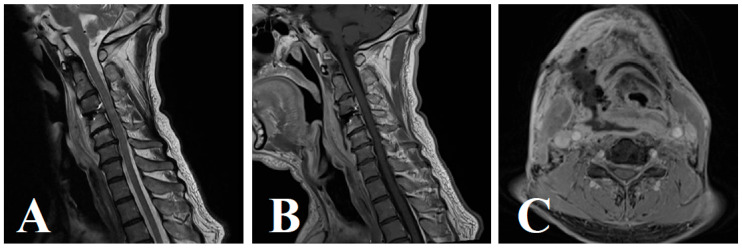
Postoperative imaging on day 7 evaluating worsening neck pain and dysphagia following C3–4 ACDF. (A) Sagittal T2-weighted MRI demonstrating a well-defined hyperintense prevertebral fluid collection anterior to the C3–4 vertebral bodies, resulting in posterior displacement of the pharynx and esophagus. This mass effect explains the patient’s progressive dysphagia. (B) Sagittal post-contrast T1-weighted MRI showing rim enhancement of the prevertebral collection, consistent with an organized abscess exerting anterior-to-posterior compression on the aerodigestive tract. (C) Axial post-contrast T1-weighted MRI confirming lateral displacement and compression of the esophagus by the enhancing collection, correlating with the patient’s swallowing difficulty and local neck discomfort.
